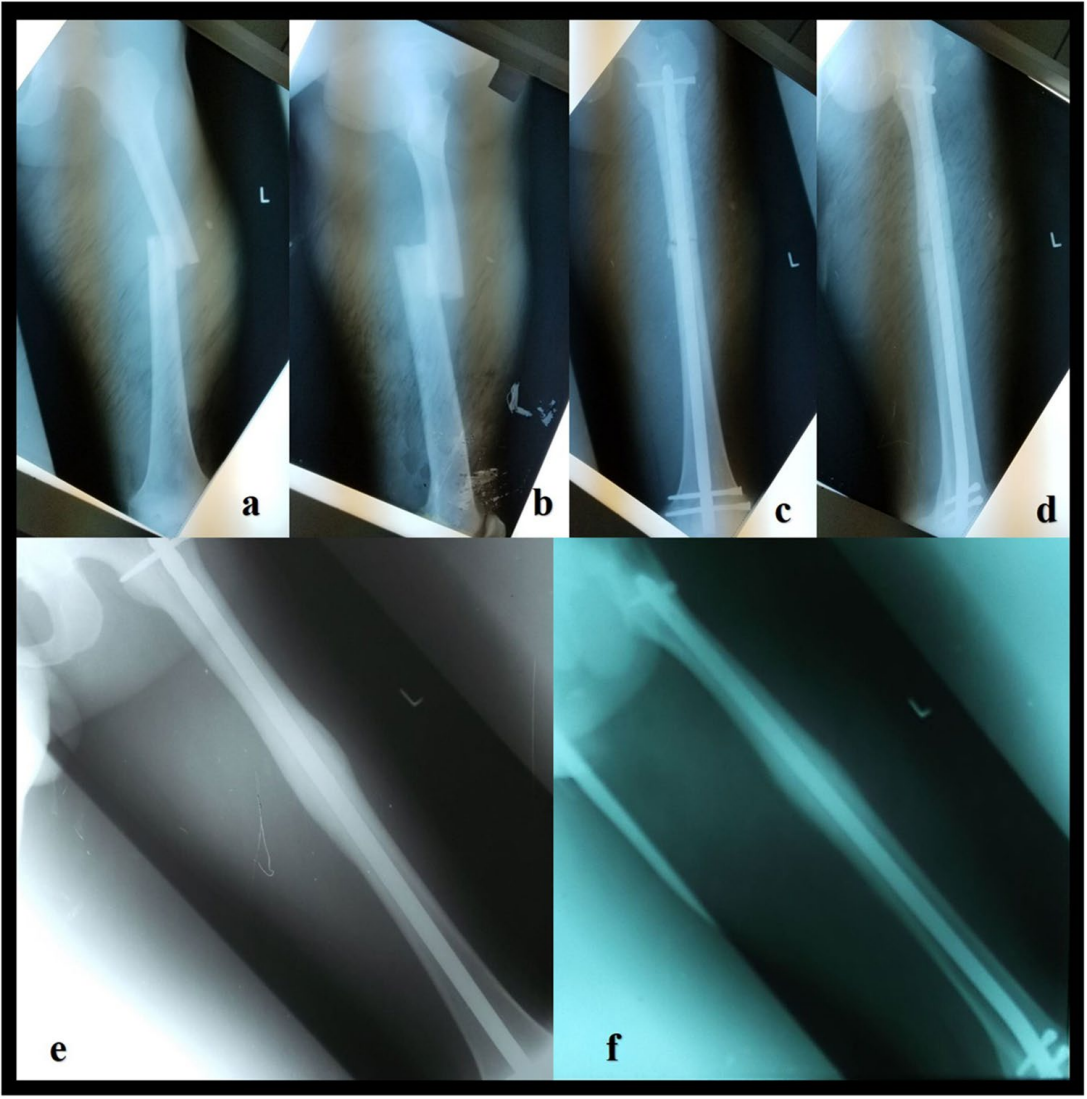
A 38 year old male patient with femoral midshaft fractures treated using SSN®, (a, b) preoperative radiographs, (c, d) postoperative radiographs, (e, f) consolidation at the follow up at 12 months
